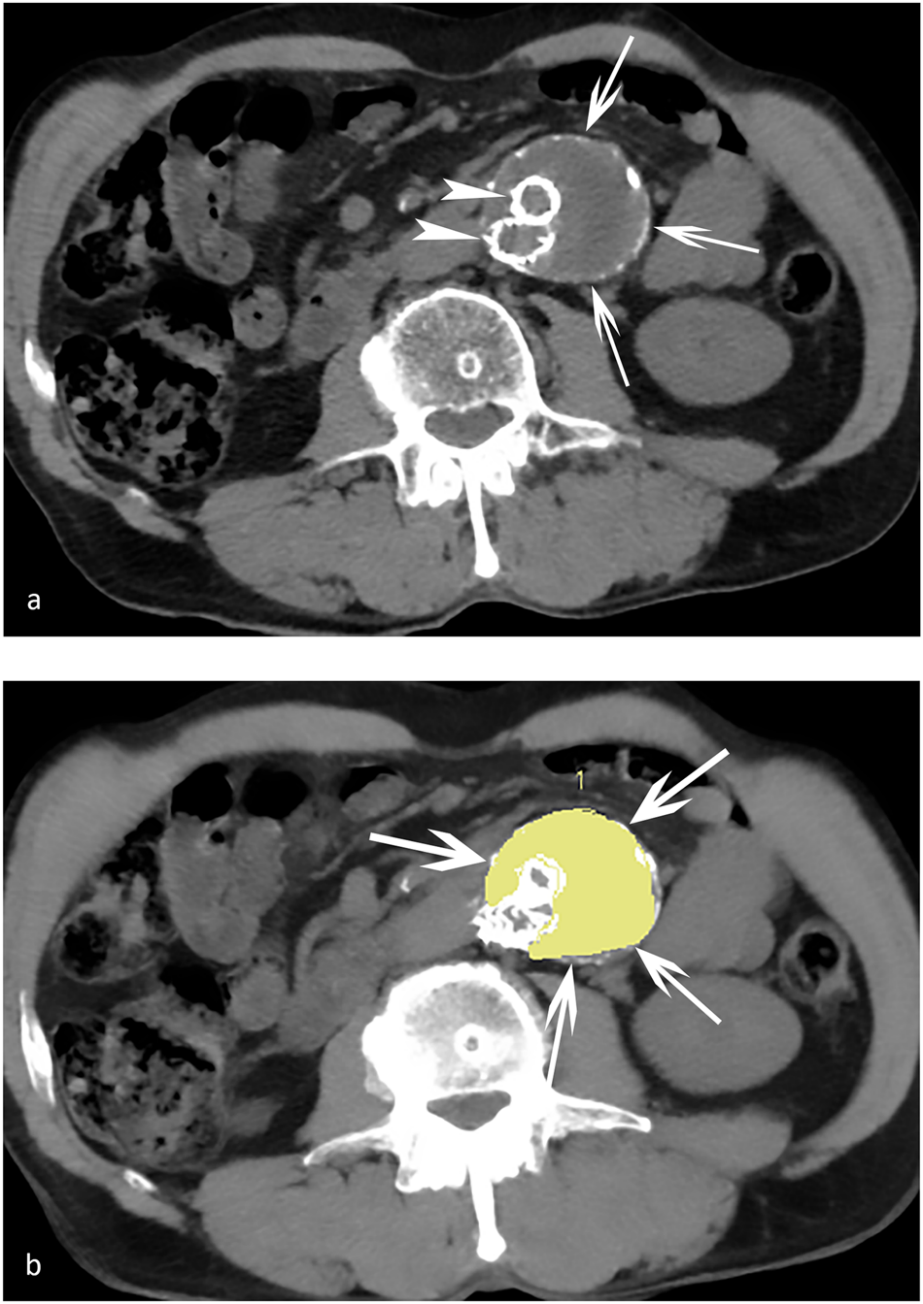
Follow-up unenhanced computed tomography one year after endovascular aortic repair. a The endograft limbs (arrowheads) within the excluded aneurysm sac (arrows) are visible. b Sac thrombus density was calculated after segmenting the sac thrombus (yellow-coloured area, arrows) on every axial computed tomography slice
